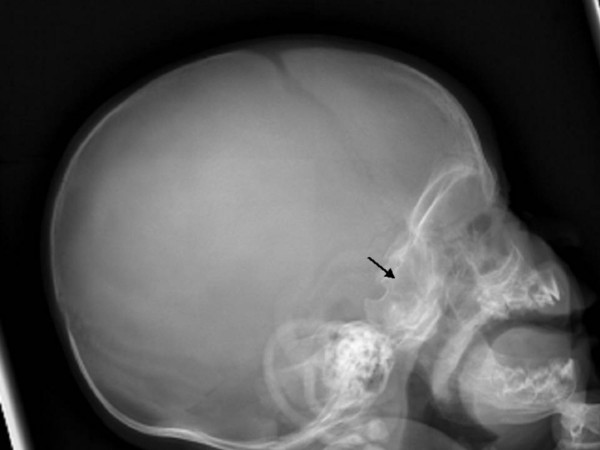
Lateral skull radiograph showed sclerosis of the skull base and hyperostosis of the calvaria (fig 2).
